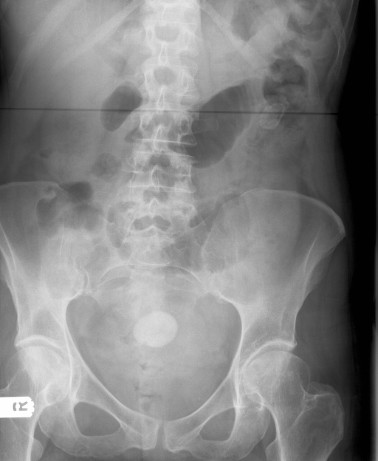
Abdominal X-ray. The X-ray shows air in the biliary tree, distended loops of small bowel, and calcified gallstone in the left side of the abdomen.
